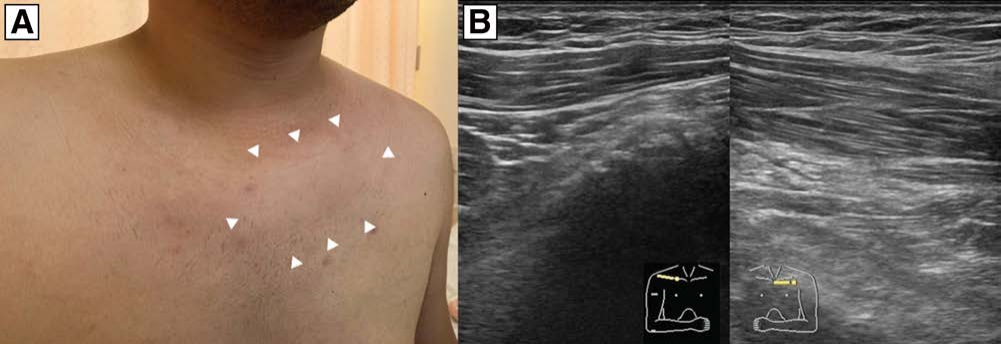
Physical examination and ultrasound findings. (A) Physical examination showed swelling of the left upper chest wall (white arrowheads) with pain triggered by left shoulder movement or deep breathing. (B) Ultrasound study revealed swollen upper intercostal soft tissue under the left pectoralis major muscle.
